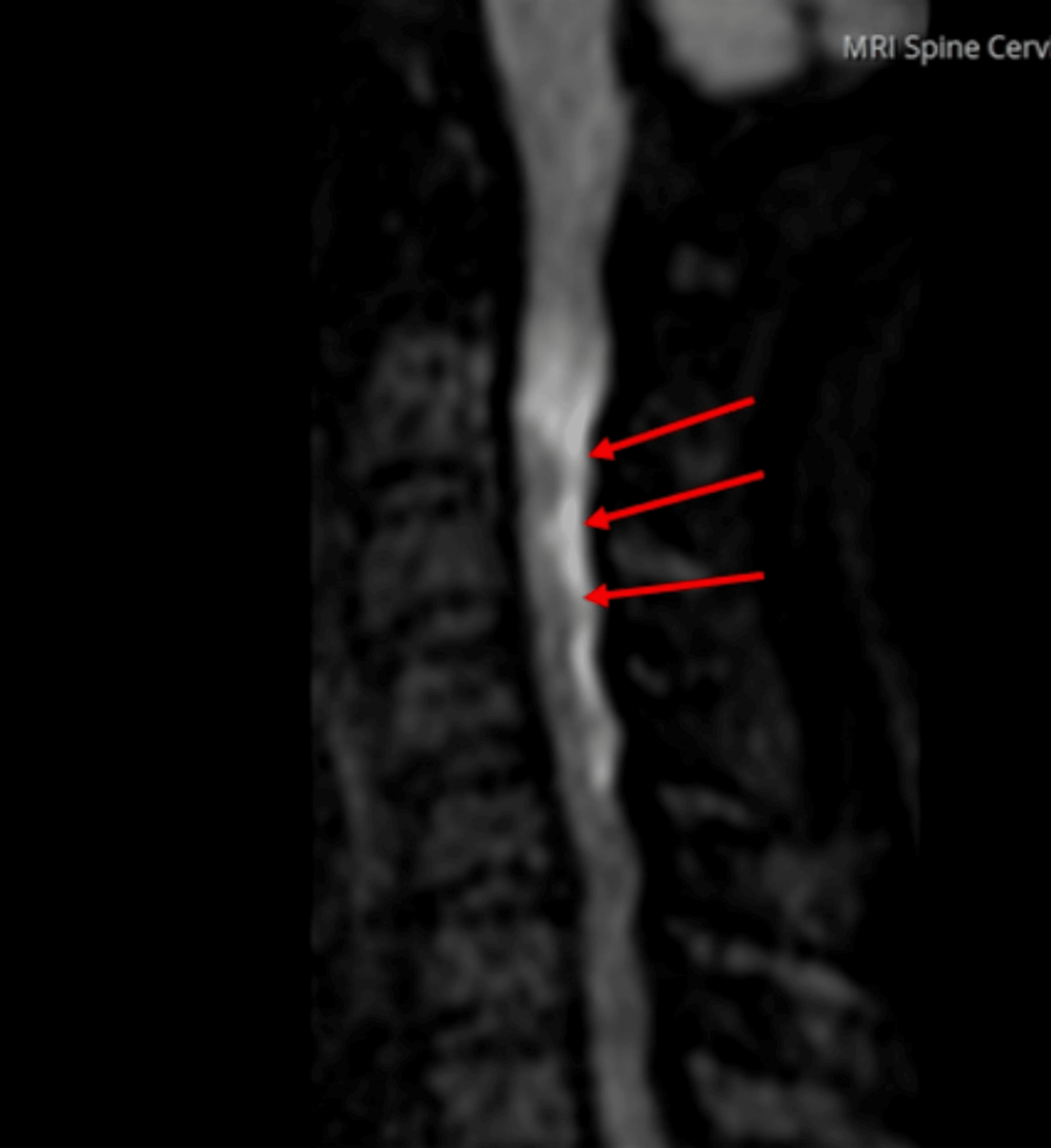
Spinal cord infarction on magnetic resonance diffusion-weighted imaging (MRI-DWI) of the cervical spine DWI of the cervical spine shows a longitudinal hyperintense signal with restricted diffusion in the posterior spinal cord extending from C2 to C5 (red arrows), consistent with acute spinal cord infarction.
